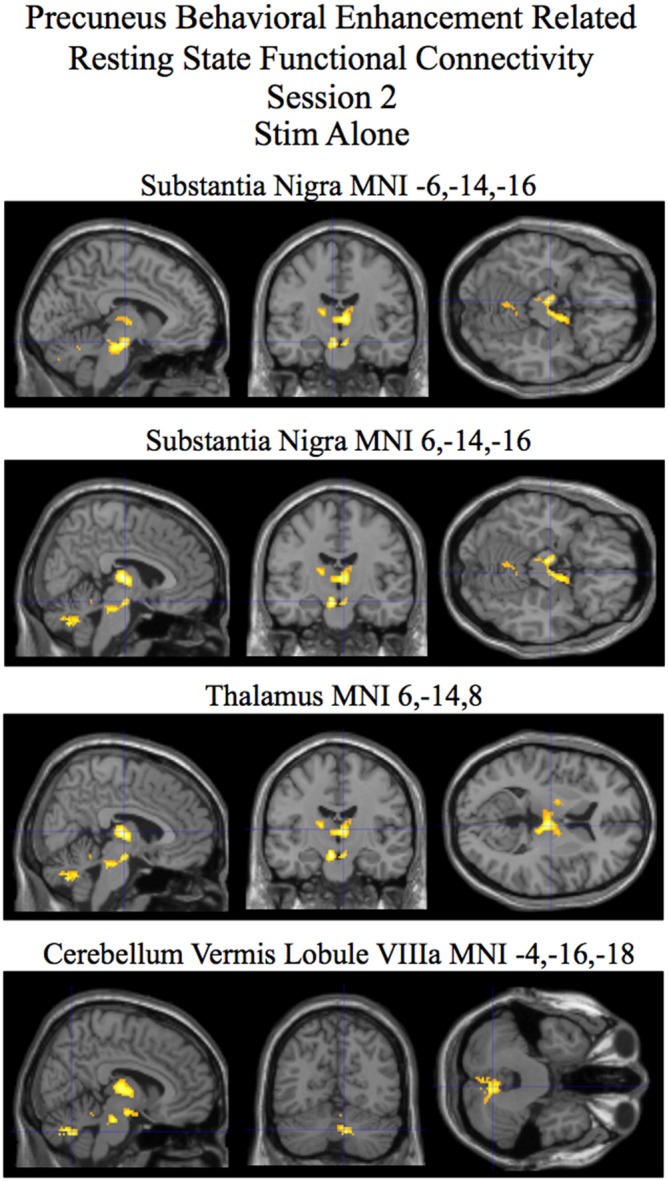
Session 2 results of the SPM random effects *t*-test for stim alone contrast for resting state functional connectivity with the precuneus using post-pre behavioral performance as a covariate of interest. Statistically significant (*p* < 0.05 corrected) behaviorally related resting state functional connectivity is rendered on sections of a template T1 MRI scan at MNI coordinates for the peaks in the various significant clusters. Negative “*x*” MNI coordinates denote left hemisphere and positive “*x*” values denote right hemisphere activity. For the MRI sections the right side of the image is the right side of the brain.
